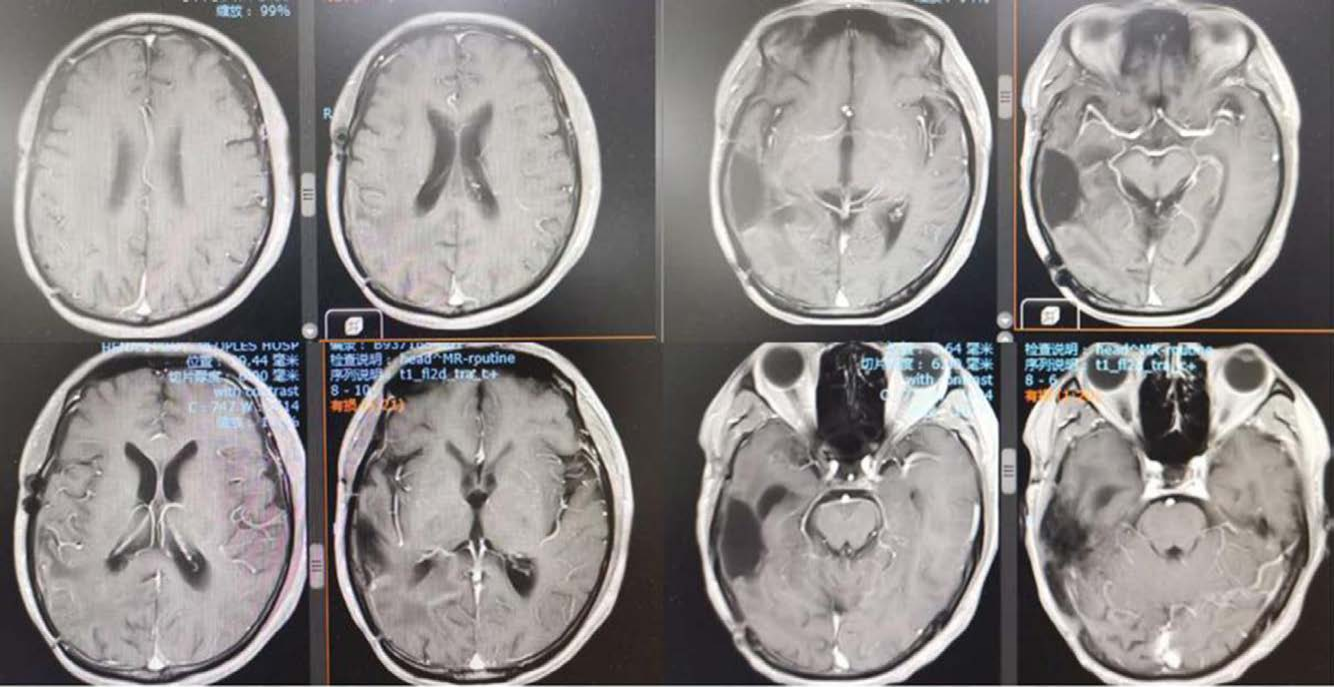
On January 10, 2017, a reexamination of the head magnetic resonance imaging showed that the condition was stable.
